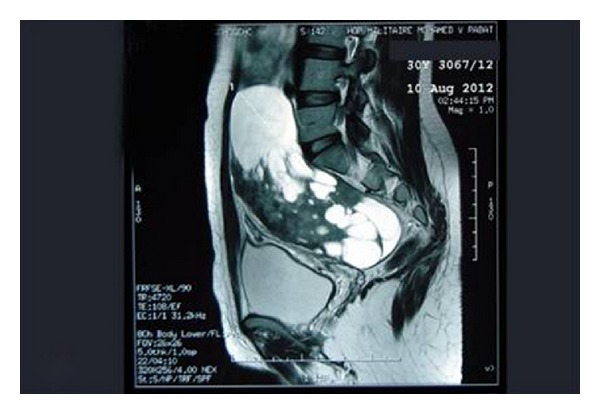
Pelvic MRI: Sagittal T2-weighted image, showing an intraperitoneal tumor with tissular and cystic signal. The tumor is separated from the uterus. The cystic areas appear hyperintense on T2-WI. And the tissular parts present heterogeneous signal on T2 sequence with hypointense areas corresponding to fibrotic tissue. The solid component of the tumor enhances strongly and heterogeneously after gadolinium injection.
